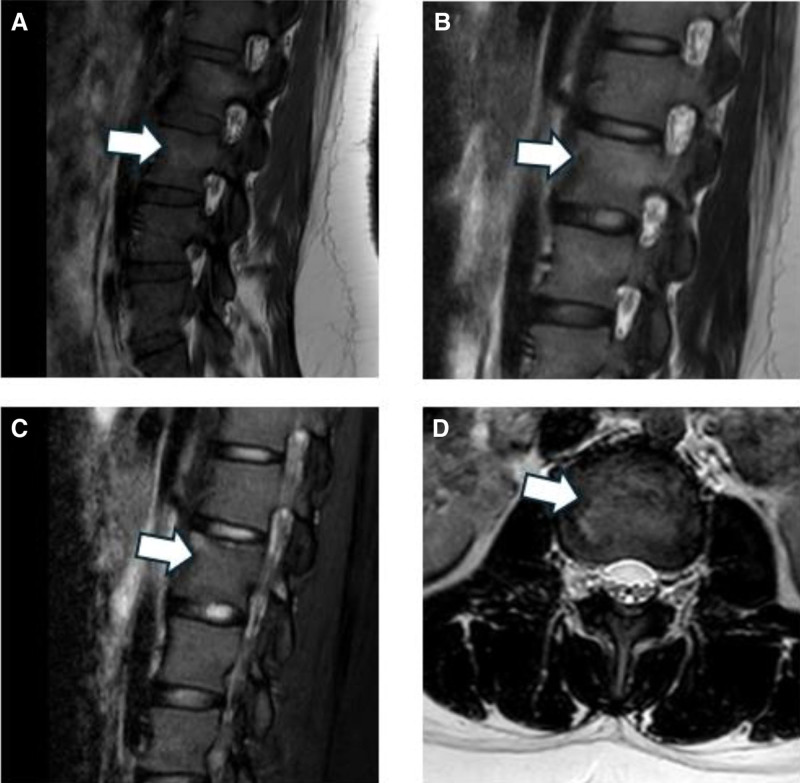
MRI images obtained after discharge nearly 1.5 yr. Sagittal T1-weighted (A), T2-weighted (B), T2-weighted fat-saturation (C), and axial T2-weighted images showed patchy abnormal signal shadows the significant reduction in the range of abnormal signals. MRI = magnetic resonance imaging.
